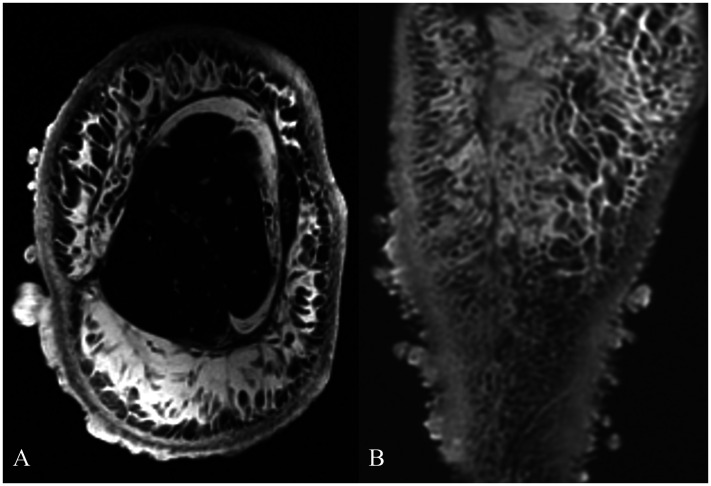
Radiologic imaging studies. A) Axial (T1-weighted image) revealing edema infiltrating subcutaneous fat and skin, extending down to the level of the deep fascia. the underlying bone and skeletal muscle show no significant alterations. B, Coronal (T1-weighted image, after injection of gadolinium contrast) revealing extensive edema with thickened skin and prominent subcutaneous vasculature. Note prominent dermal nodules.
